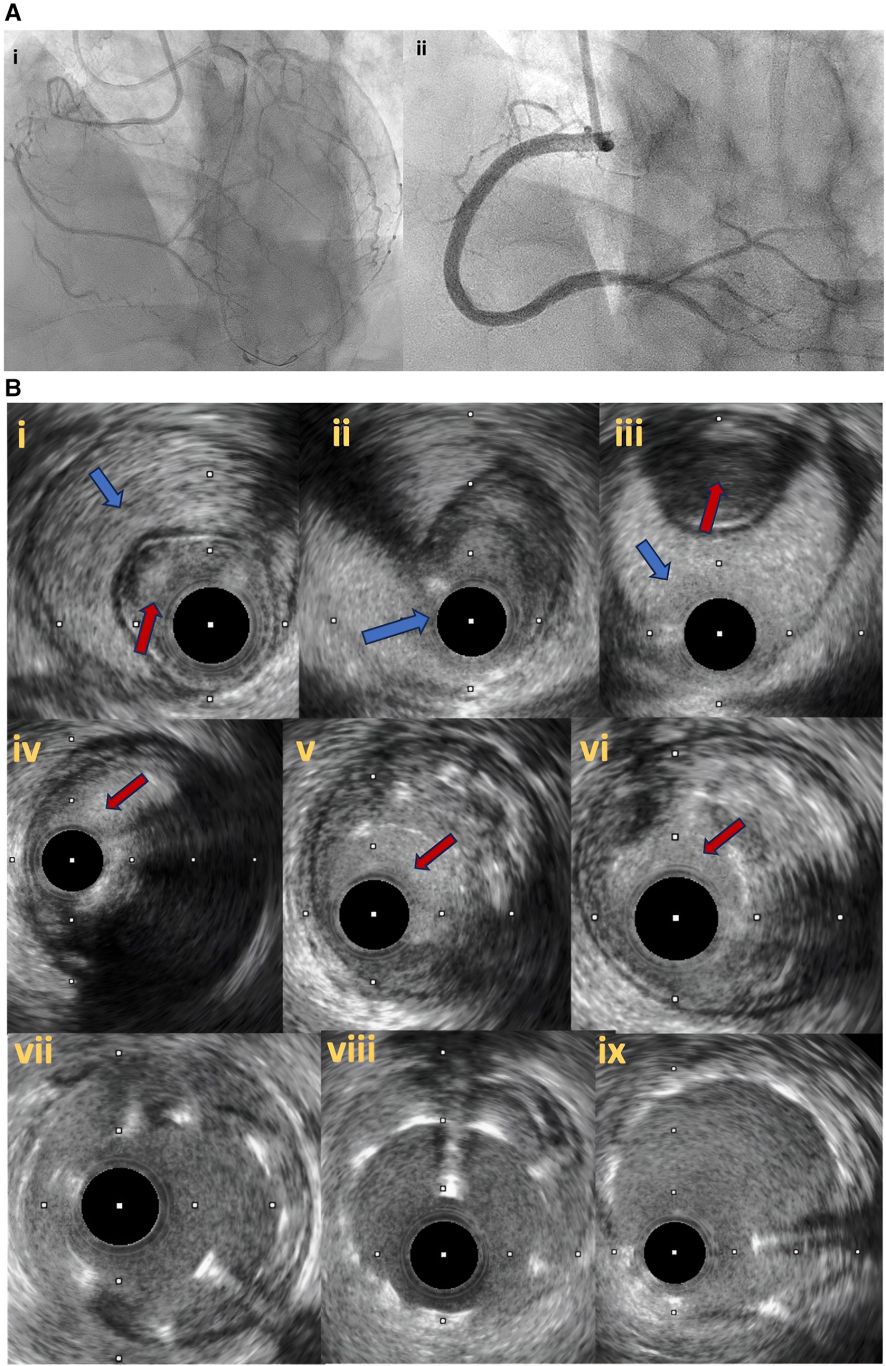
(A) Right coronary artery total occlusion revascularization with antegrade dissection reentry technique (7Ai–ii). (B) Intravascular ultrasound pictures after successful distal true lumen reentry and CTO recanalization. In (7Bi), intraluminal wire position (red arrow) with large subintimal haematoma is noticed. In (7Bii), the transition from true lumen into subintimal space is noticed (blue arrow), whilst in (7biii) the wire is in the subintimal space (blue arrow) that compresses the true lumen (red arrow). In (7Biv–vi), intraplaque wire tracking is noticed. Final IVUS images after stent deployement (7bvii–ix).
